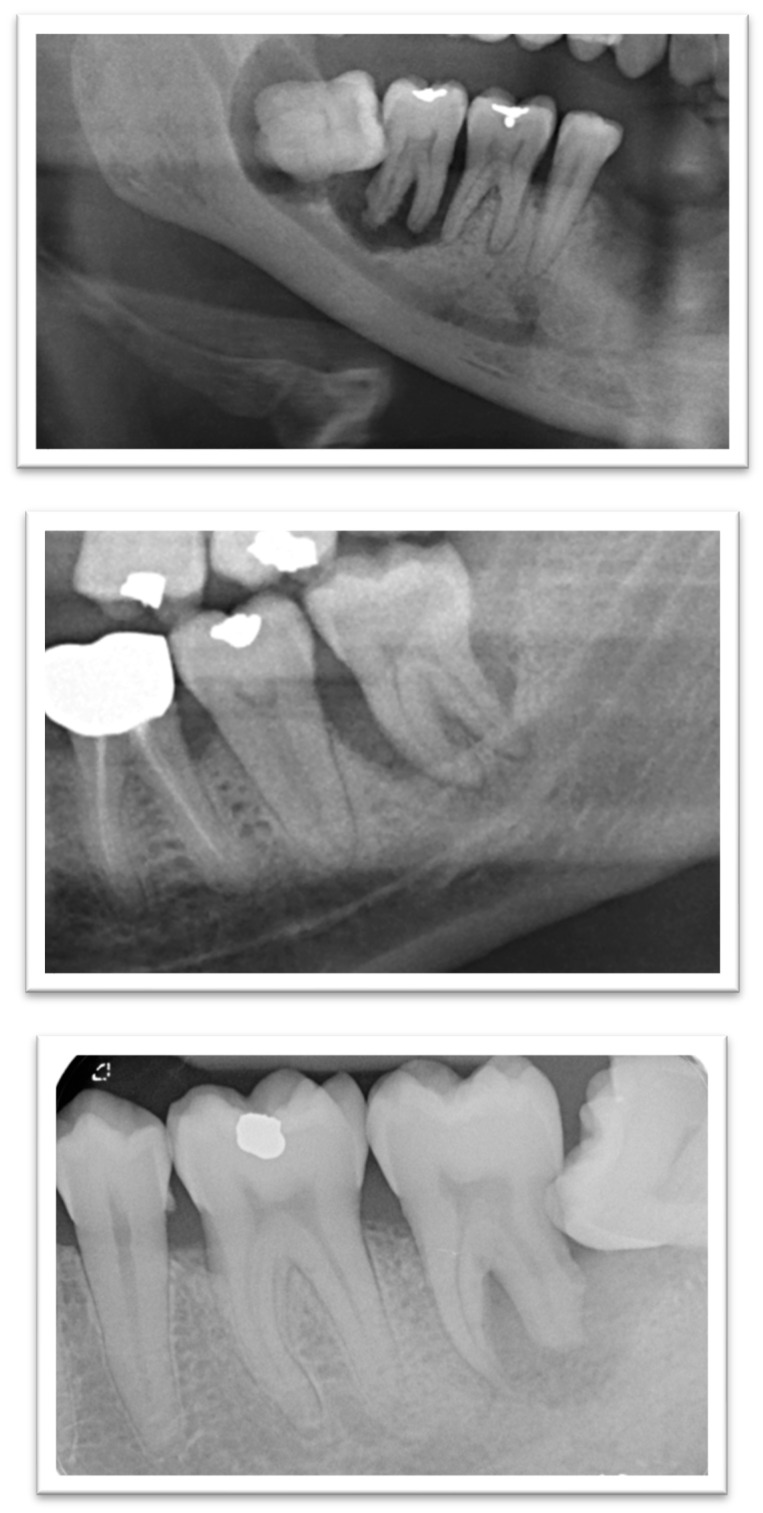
Radiographs of excluded cases with perio-endo lesions. These cases interfered with the assessment of intrabony defects due to the presence of perio-endo lesions, leading to the exclusion of three 2Ms from the final analysis.
